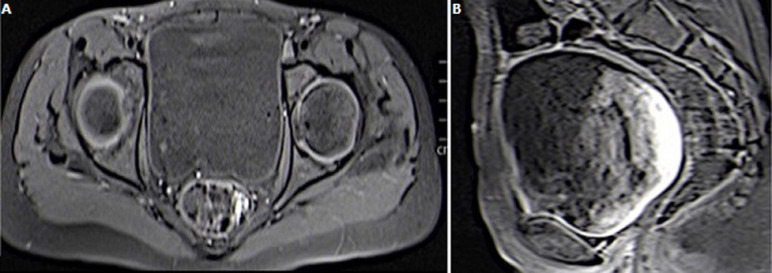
MRI 3 months after the surgery showed absence of centropelvic mass
